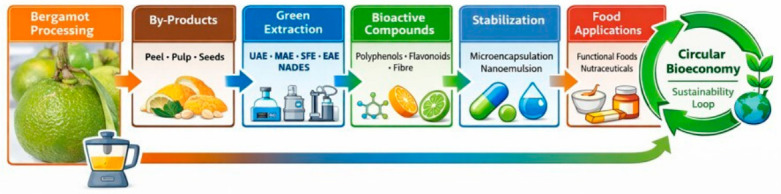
Integrated valorisation pathway of bergamot by-products. Abbreviations: UAE, ultrasound-assisted extraction; MAE, microwave-assisted extraction; SFE, supercritical fluid extraction; EAE, enzyme-assisted extraction; NADES, natural deep eutectic solvents.
